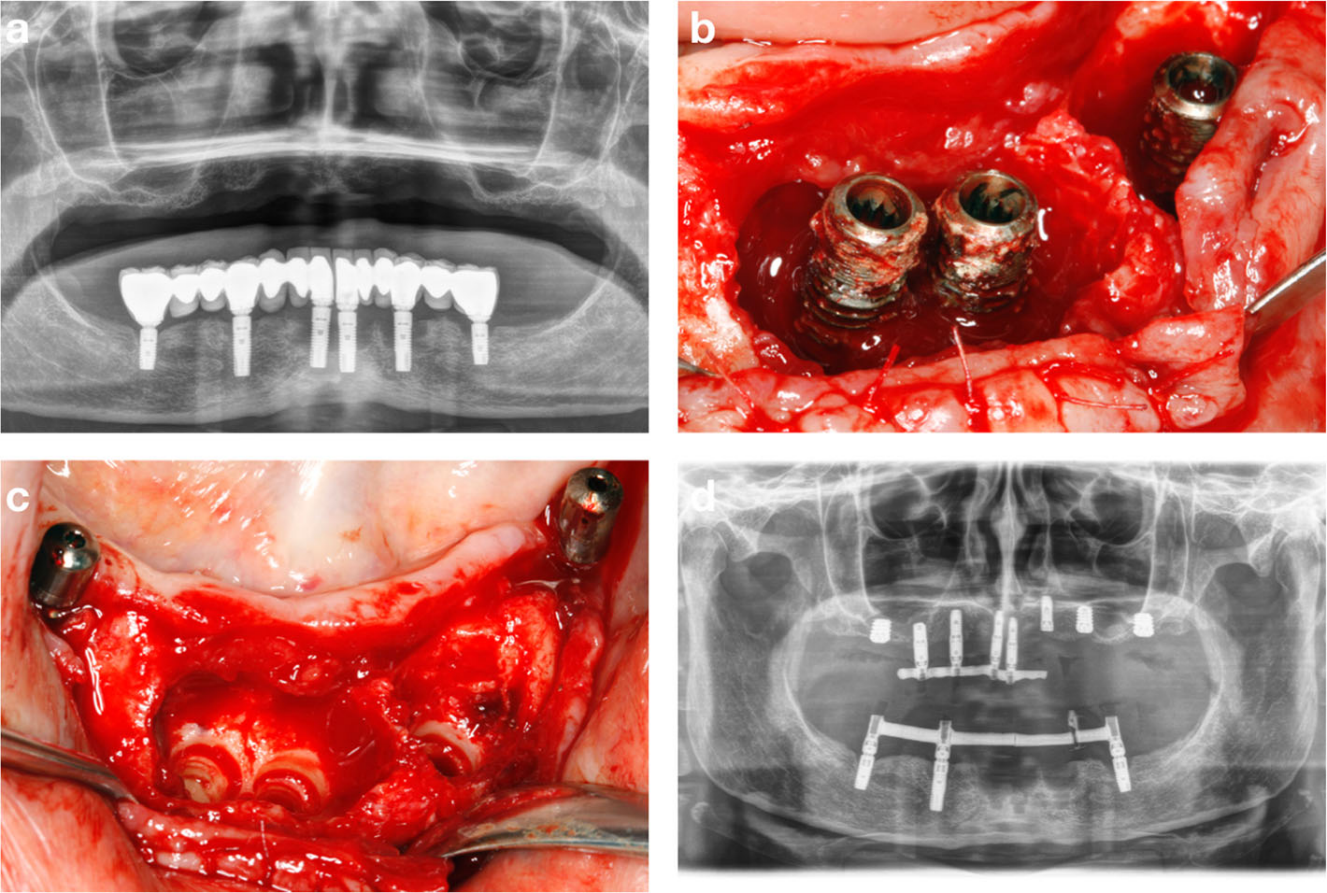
Panoramic radiograph showing excessive marginal bone loss affecting all the dental implants in the mandible supporting fixed prostheses (a). Clinical image showing the advanced bone destruction around the implants at the incisors and left premolar regions (b). Clinical image showing the preservation of the pre-existing bone upon implant removal with the counter-torque regions (c). Panoramic radiograph showing the maintenance at this stage of 3 implants to support the provisional prosthesis in the mandible (d)
